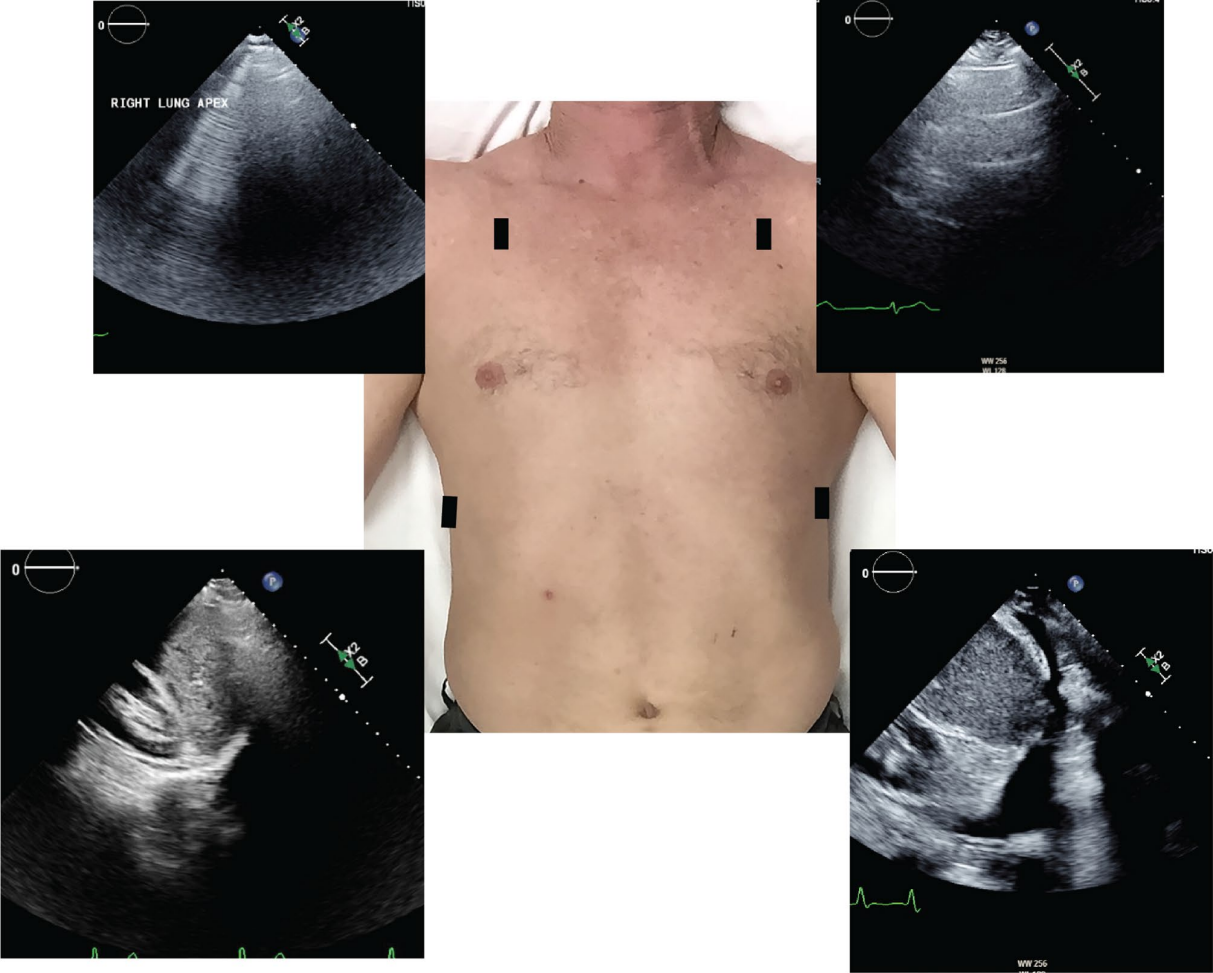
4-view lung ultrasound exam: Lung ultrasound exam included 2D imaging only in the longitudinal (parasagittal) view in the lung apex (intercostal space #3, mid-clavicular line) and posterior coronal views of the lower lobes at the diaphragm. Orientation of the image maintains the cardiac convention, cephalad and directional markers on the right of the screen, for seamless acquisition by the sonographer. Sample B-lines (top-left) and pleural effusion (bottom-right) shown
